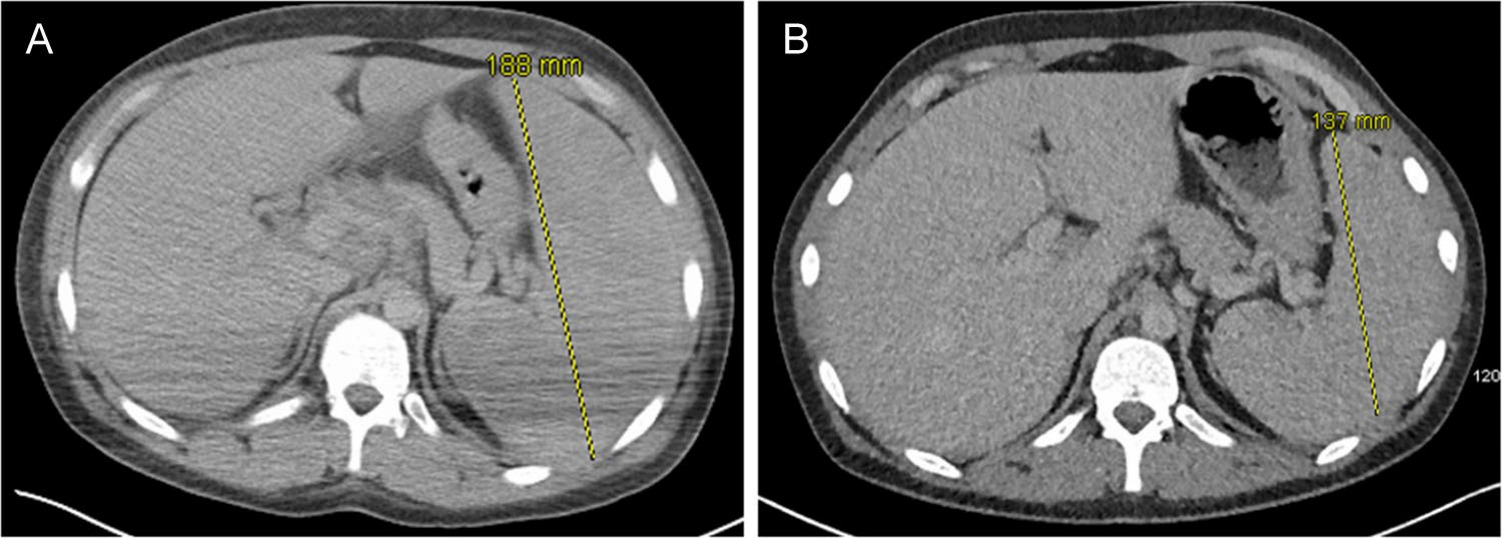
Axial CT images of the abdomen pre- (A) and 18 days post-treatment (B). Splenomegaly, with mediastinal, porta hepatis and para-aortic lymph node enlargement is demonstrated in A and its significant reduction in B. Splenic transverse diameter (yellow line) and corresponding measurement shown
